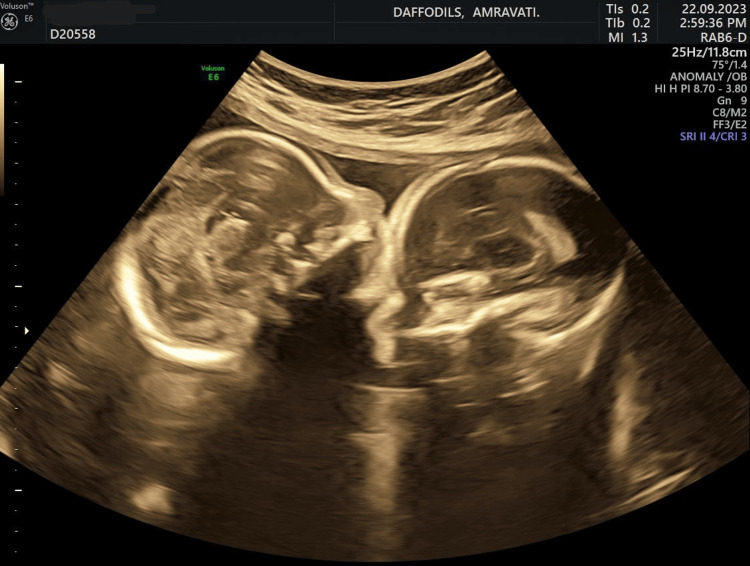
USG scan of 19-week foetuses, transabdominal route USG: ultrasonography
